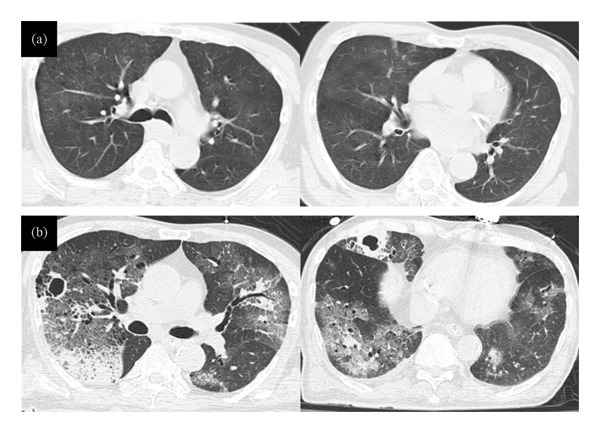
Changes in the imaging findings on chest CT scan. On admission, widespread pale ground‐glass opacities were observed in both lungs due to water aspiration (a). Ground‐glass opacity and infiltrating shadows were identified mainly around the bilateral airways on Day 11. New hollow shadows were also noted in the right upper and middle lobe on Day 11 (b). Left: images of the upper lobes; right: images of the middle and lower lobes.
